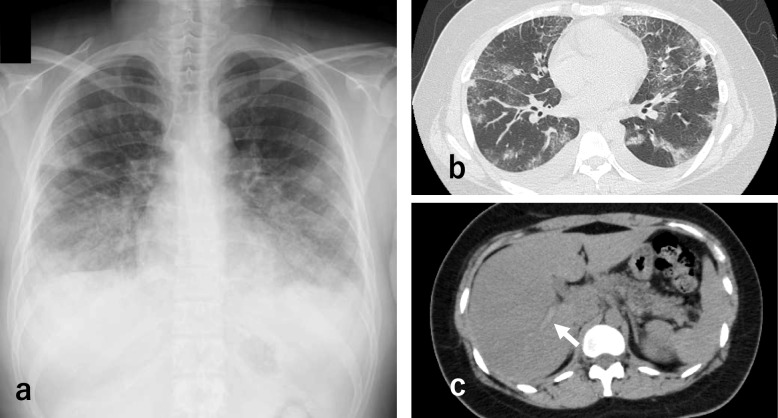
Image findings on admission (day 1). a Chest radiograph showing bilateral infiltrative shadows. b Chest computed tomography (CT) scan showing bilateral ground-glass opacities and bilateral pleural effusion. c Abdominal CT scan showing a portal vein thrombosis
